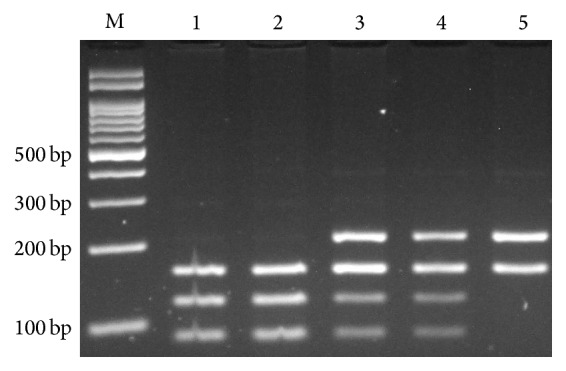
Electrophoresis pattern of PCR-RFLP for detection of* IFNGR1* rs7749390 polymorphism. The 366-bp PCR product digested by BstC8I. C allele produces 87, 121, and 158 bp, while the T allele produces 208 and 158 bp. M: DNA marker; lanes 1 and 2: CC; lanes 3 and 4: CT; Lane 5: TT.
